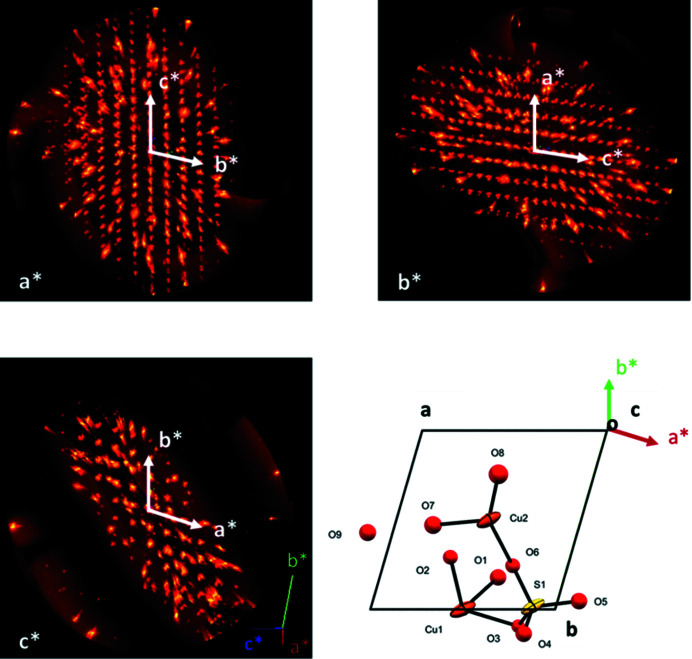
Reconstructed X-ray precession plots viewed along *a, *b and *c. The bright spots exhibiting radial elongation arise from the diamond anvils. The view along the *c axis clearly shows dark areas of reciprocal space due to the shielding of the mini-DAC. A view of the asymmetric unit of copper sulfate pentahydrate along the *c* axis shows the Cu—O and S—O bonds, with the H atoms removed from the structure for clarity, and helps to identify those bonds which have their projections predominantly in the *a*b plane.
